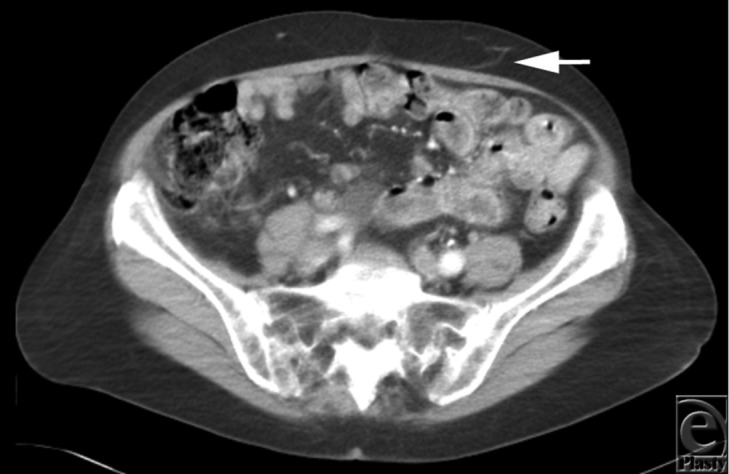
Case 1 - Left sided perforator vessel supplying overlying skin and fat (arrow) on CT angiogram.
